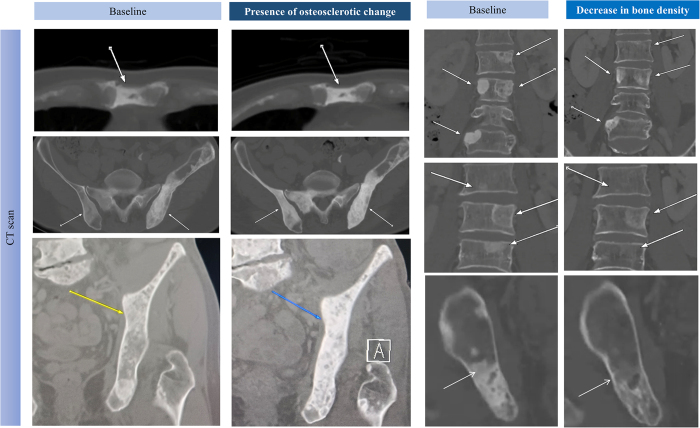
Typical image on assessment of osteosclerotic changes (OCs), and no osteosclerotic changes (NOC) in prostate cancer with osteoblastic metastasis. CT attenuation of the lesion increased over 10% in bone lesions defined as OC, otherwise NOC. Besides, in three cases with OCs, the radiography progression-free survival (rPFS) was 1.4, 14.0, and 9.1, respectively. In three cases with NOCs, the rPFS was 26.5, 10.0, and 30.4, respectively.
